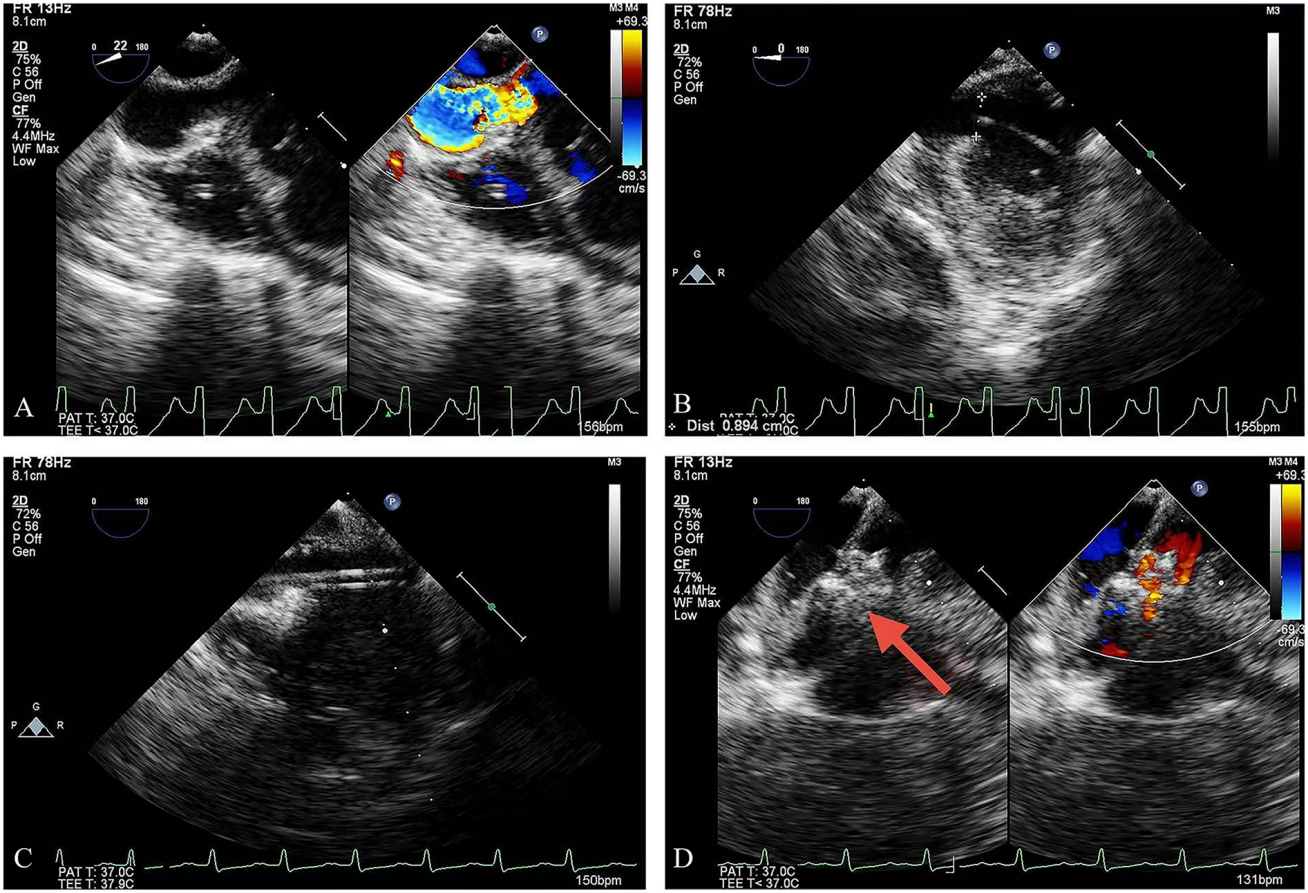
TEE guidance: (A) the outlet of the CAF; (B) the guidewire was advanced into the LV; (C) the delivery sheath was introduced over the guidewire; (D) deployment of the occlusion device (red arrow = device). TEE, transesophageal echocardiography; LV, left ventricle.
